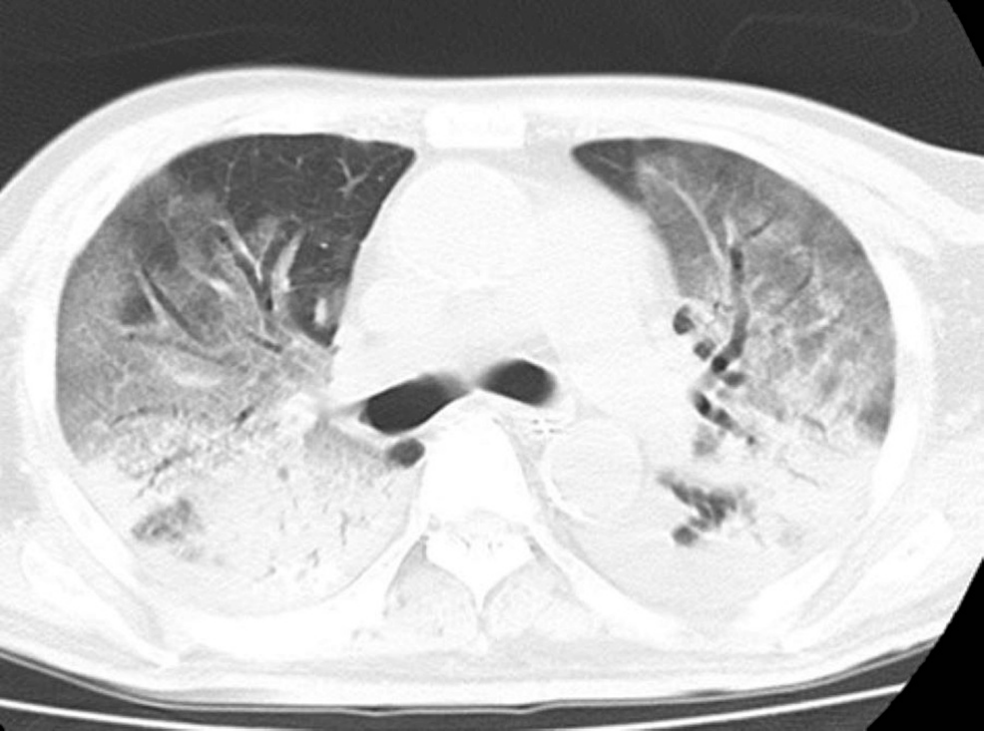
Follow-up unenhanced CT on day 10 The CT scan showed an increase in ground glass opacity density in both lungs CT: computed tomography
